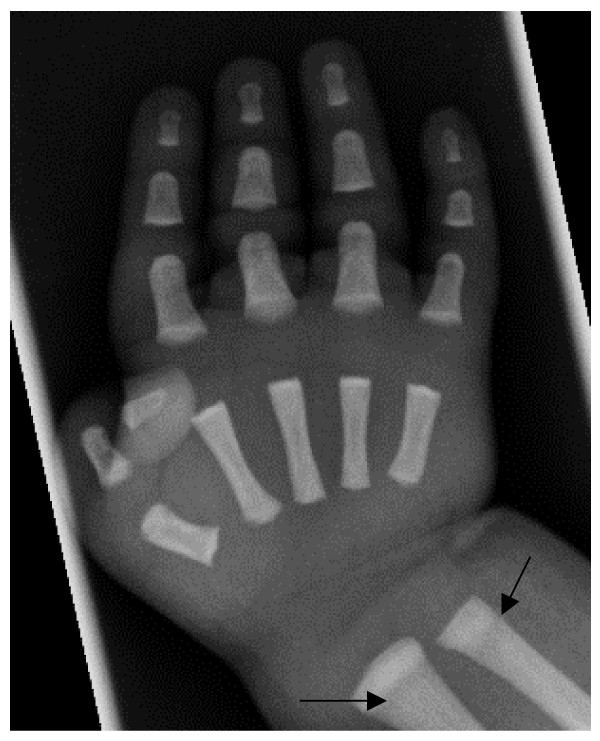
Severe ARO: Right hand radiograph, age 2 weeks. Note metaphyseal lucent bands in the distal ulna and radius (arrows) and short tubular bones.
